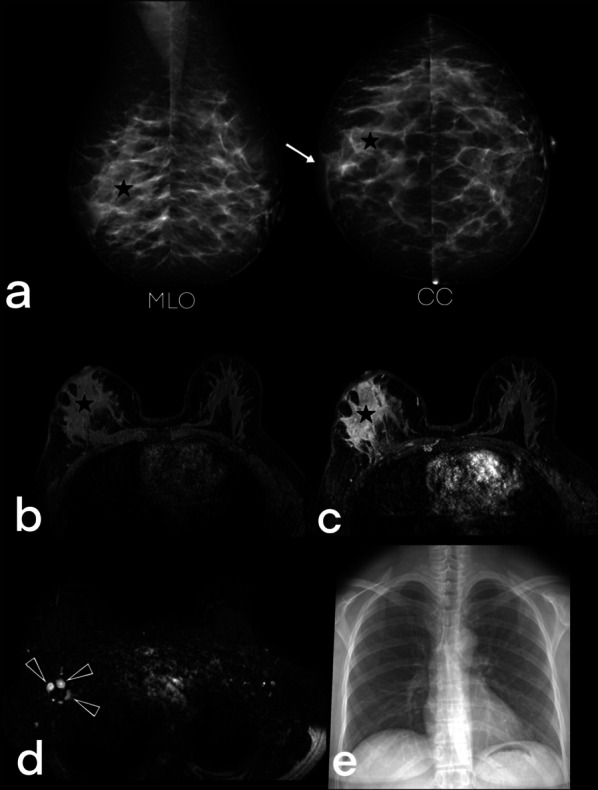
Mammograms of a 44-year-old female demonstrate disseminated tuberculosis in right breast (a) show an asymmetric opacity (star) and thickening of the skin (arrow). MRI images of the same patient: pre-contrast fat-suppressed T1-weighted (b), post-contrast fat-suppressedT1-weighted (c), and fat-suppressed T2-weighted images (d) show homogeneous non-mass enhancement with regional type distribution (star) and axillary lymph nodes (arrowheads). The chest x-ray (e) of the patient is negative for pulmonary tuberculosis. BIRADS 4
